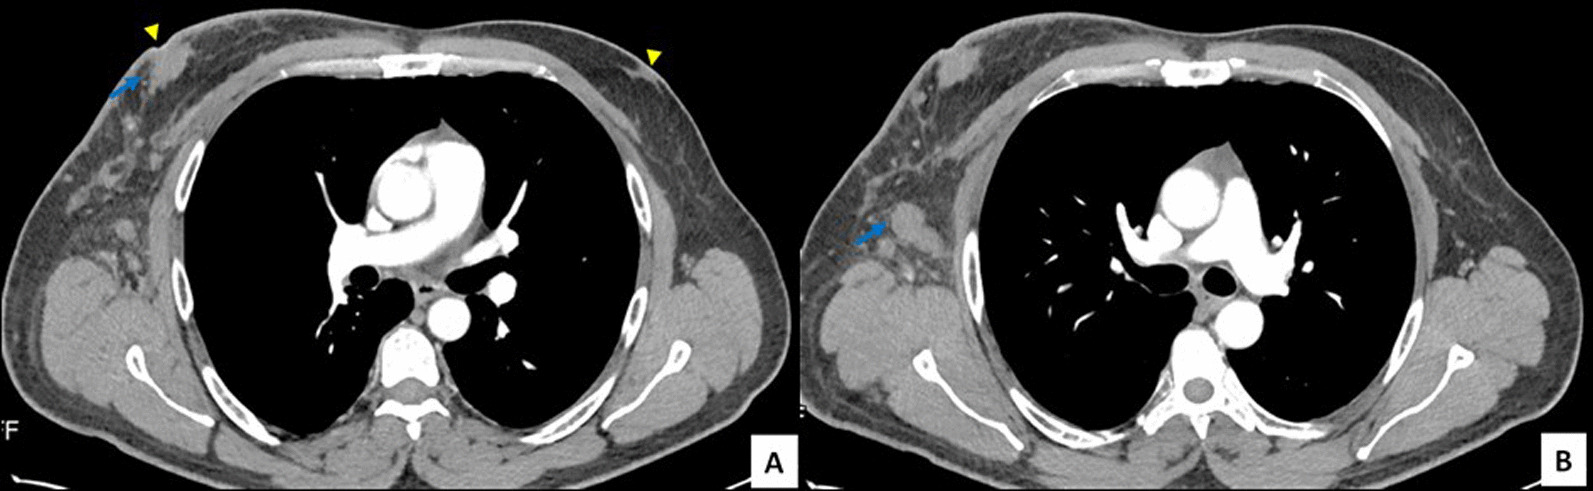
Arterial phase thorax CT (mediastinal window axial cuts).  A demonstrates bilateral gynecomastia (arrowheads) with enhancement right retroareolar mass (blue arrow) and B ipsilateral lymph node enlargement (blue arrow)
